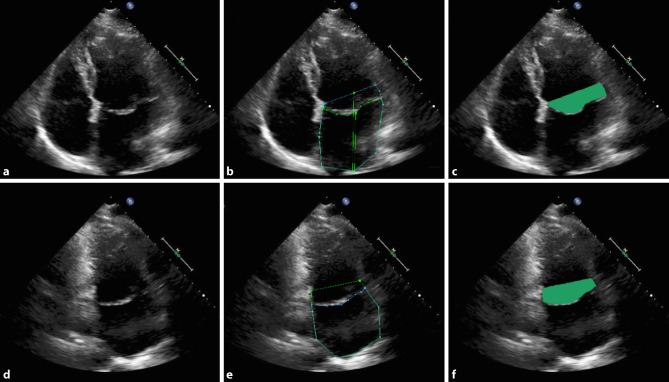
a–f Echocardiographic evaluation and quantification of mitral prolapsing volume. a–c Four-chamber view demonstrating a mitral annular disjunction, b measurements of atrial dimensions up to the level of the annulus (*blue*) and the mitral valve (*green*), resulting in c the prolapse surface. d–f Two-chamber view in the same patient demonstrating d mitral annular disjunction, e measurements and f prolapse surface
